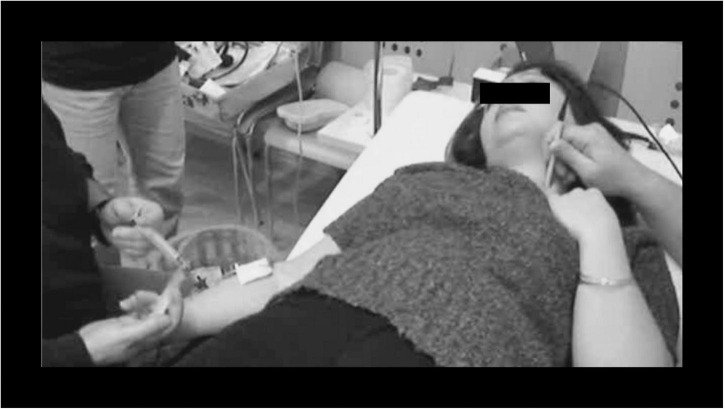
Carotid Doppler test. Right arm: injection of contrast medium (10 mL of agitated saline solution); Left carotid artery: 8 MHz Doppler probe.
